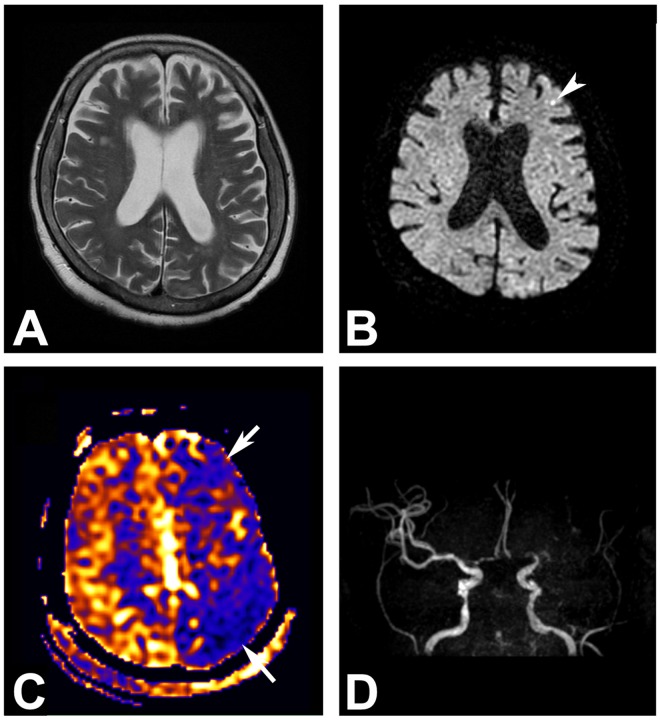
A 87-year-old woman who showed a convulsive seizure in the patient ward. (A) Brain parenchyma appears normal on the T2-weighted image. (B) A tiny dot-like diffusion restriction is noted at the left frontal cortex (arrowhead). Subtle cortical hyperintensities at the left occipital lobe are artifacts. (C) Arterial spin labeling perfusion-weighted MR image depicts hypoperfusion at the left middle cerebral artery territory (arrows). (D) MR angiography shows occlusion at the left M1 segment.
